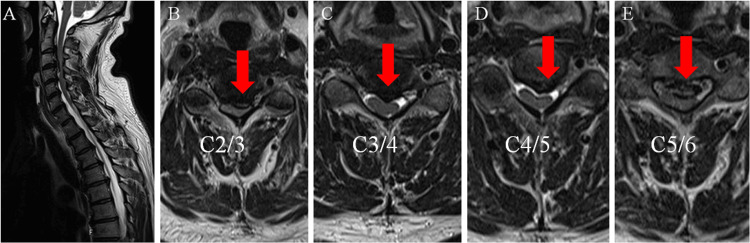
MRI of the cervical spine at the initial examination (A) MRI of the midsagittal section; (B-E) MRI of the horizontal section. Spinal canal stenosis is seen at C2/3, C3/4, C4/5, and C5/6 (red arrows).
